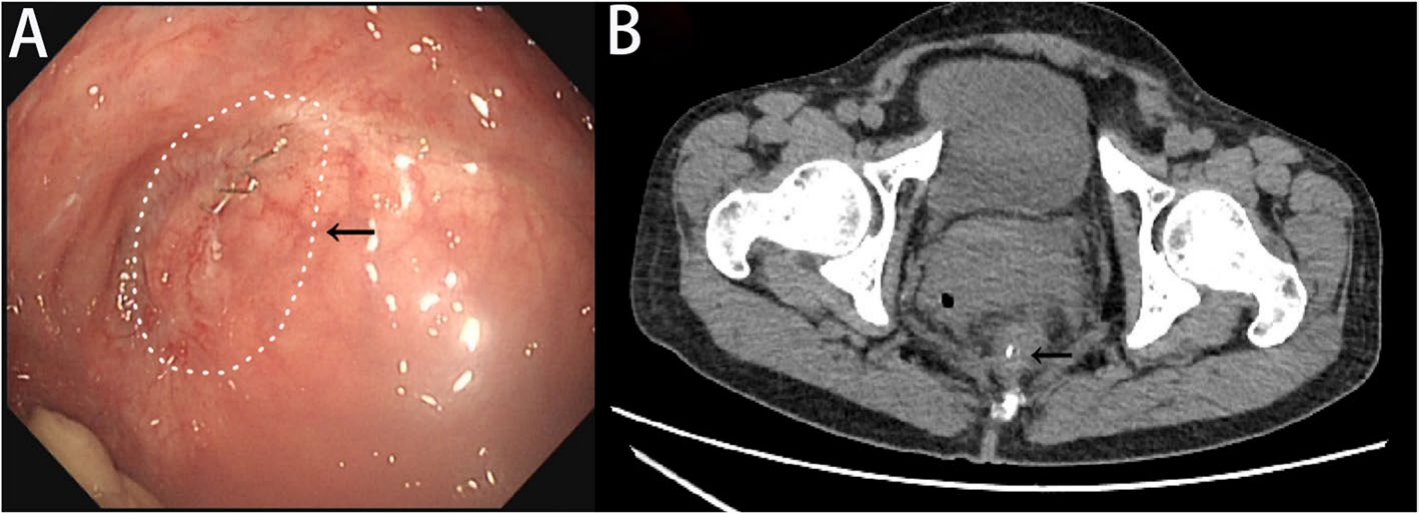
Colonoscopy and abdominal CT examination revealed that the rectal anastomosis was already occluded, and some anastomotic metal nails were observed at the stoma site
